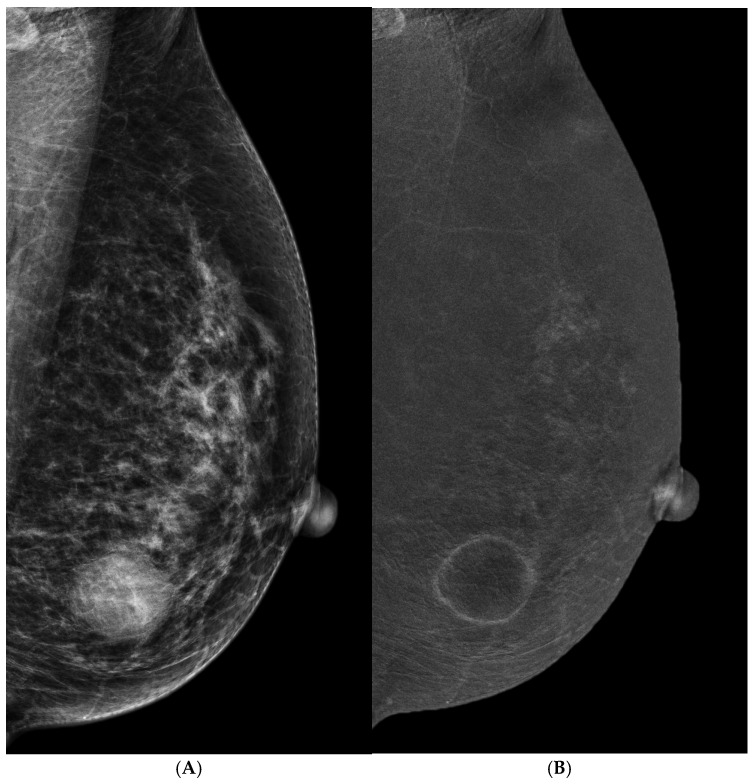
A 52 y/o woman with a palpable mass in the lower inner quadrant of the left breast. (A) LM (MLO view) showed a round circumscribed hyperdense mass in the lower region of the left breast. (B) REI (MLO view) showed a thin and smooth rim enhancement without internal enhancement, which was compatible with a simple inflamed cyst. The sonography of the breast also demonstrated a pure simple cyst.
